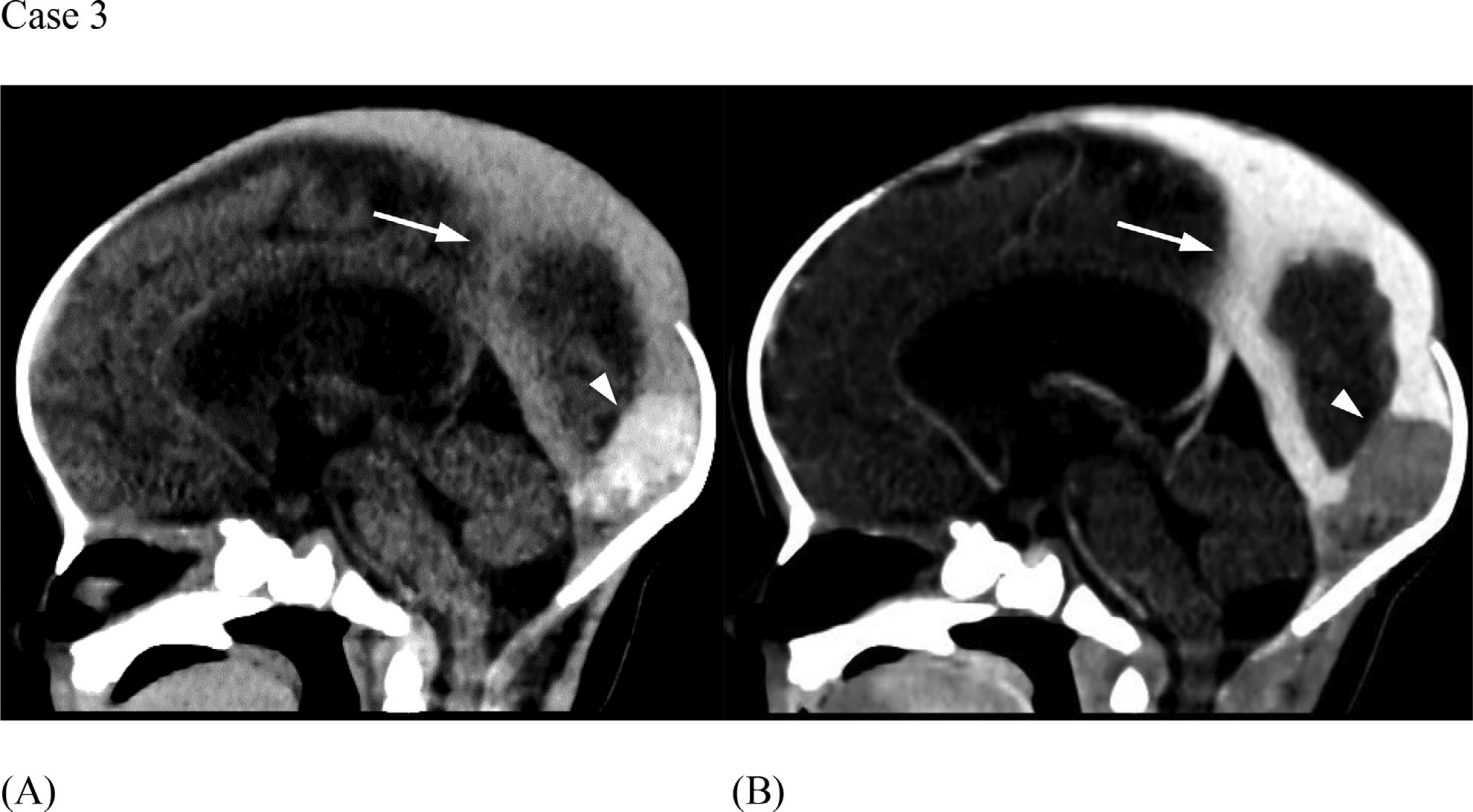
(Case 3) Sagittal CT without contrast (A) and with contrast (B) demonstrate persistent falcine sinus (arrows) and superior sagittal sinus leading to torcular thrombus (arrowheads), likely formed due to irregular flow dynamics.
